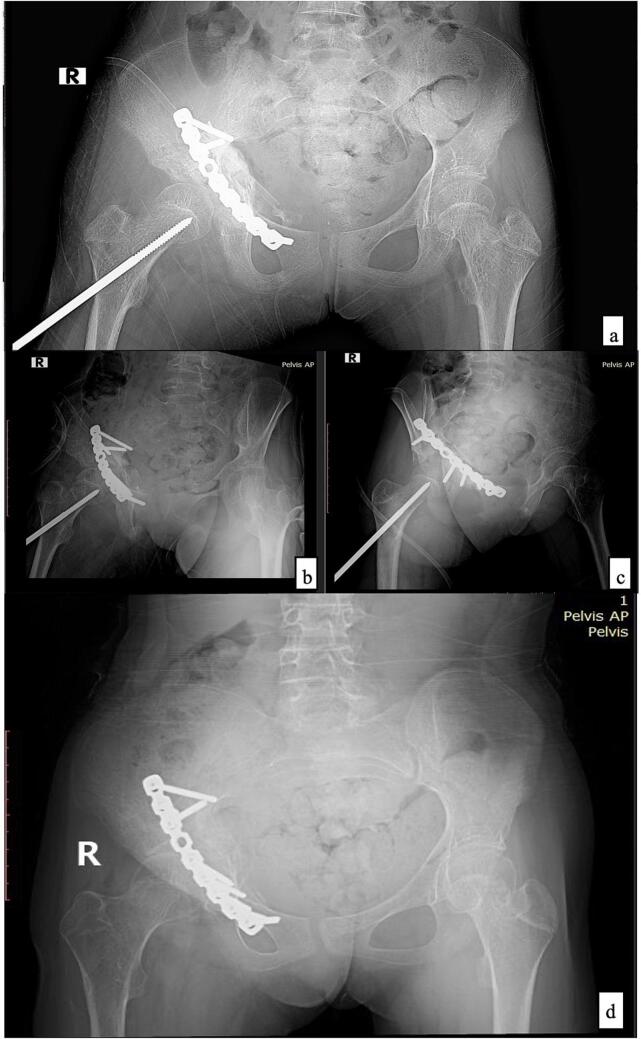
Post-operative radiological examination of pelvis X-ray: (a) AP view, (b) right iliac oblique view, (c) right obturator oblique view, (d) pelvis AP view follow-up evaluation post skeletal traction removal.
